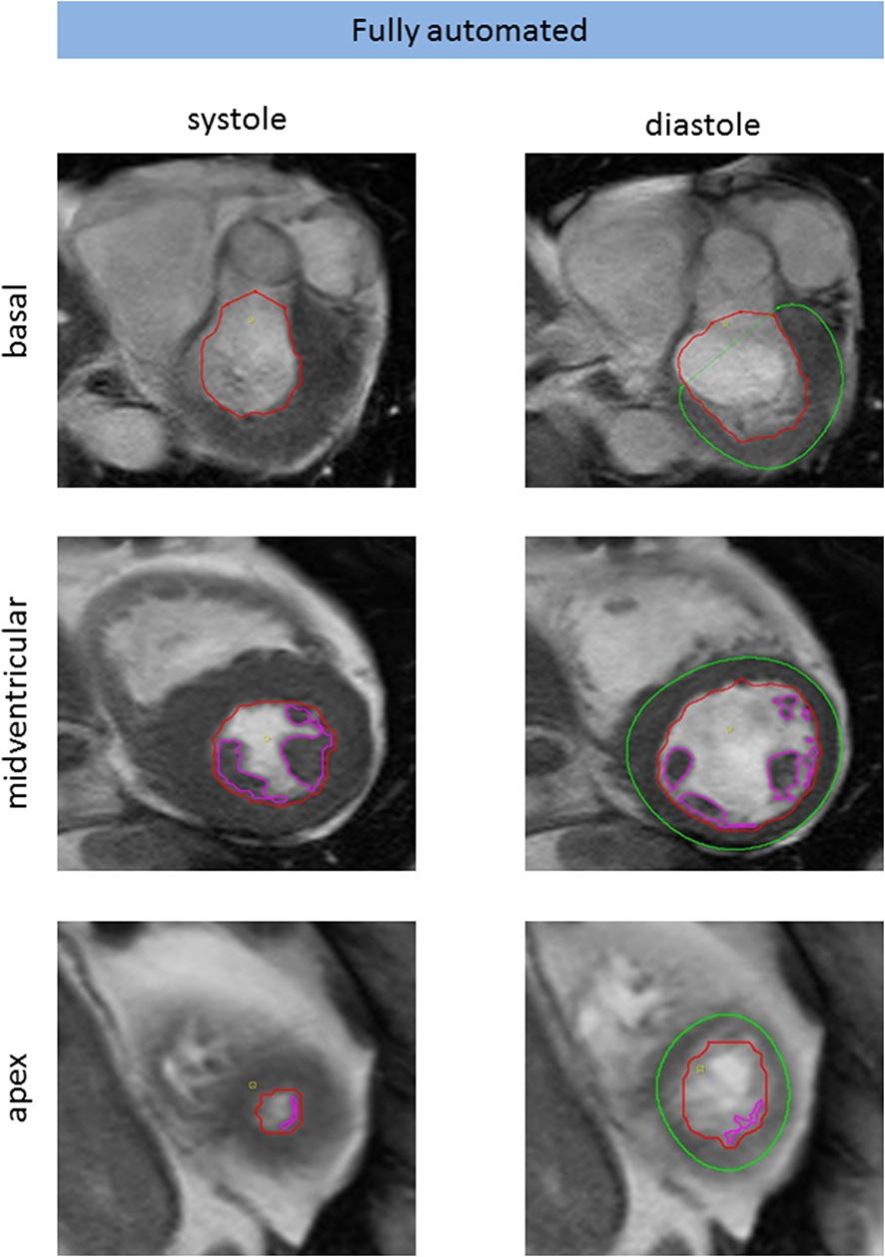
Representative example of accurate fully automated contouring. Short axis cine MRI sequence of a 77-year old man with no pathological findings. Visualized are the fully automated generated contours of apex, midventricular and basal slices in systole (left) and diastole (right). Contours are shown in red (subendocardial), pink (papillary muscles) and green (subepicardial). No manual corrections were necessary in this case. LV ejection fraction was 65.3% for both fully automated and expert corrected analysis
